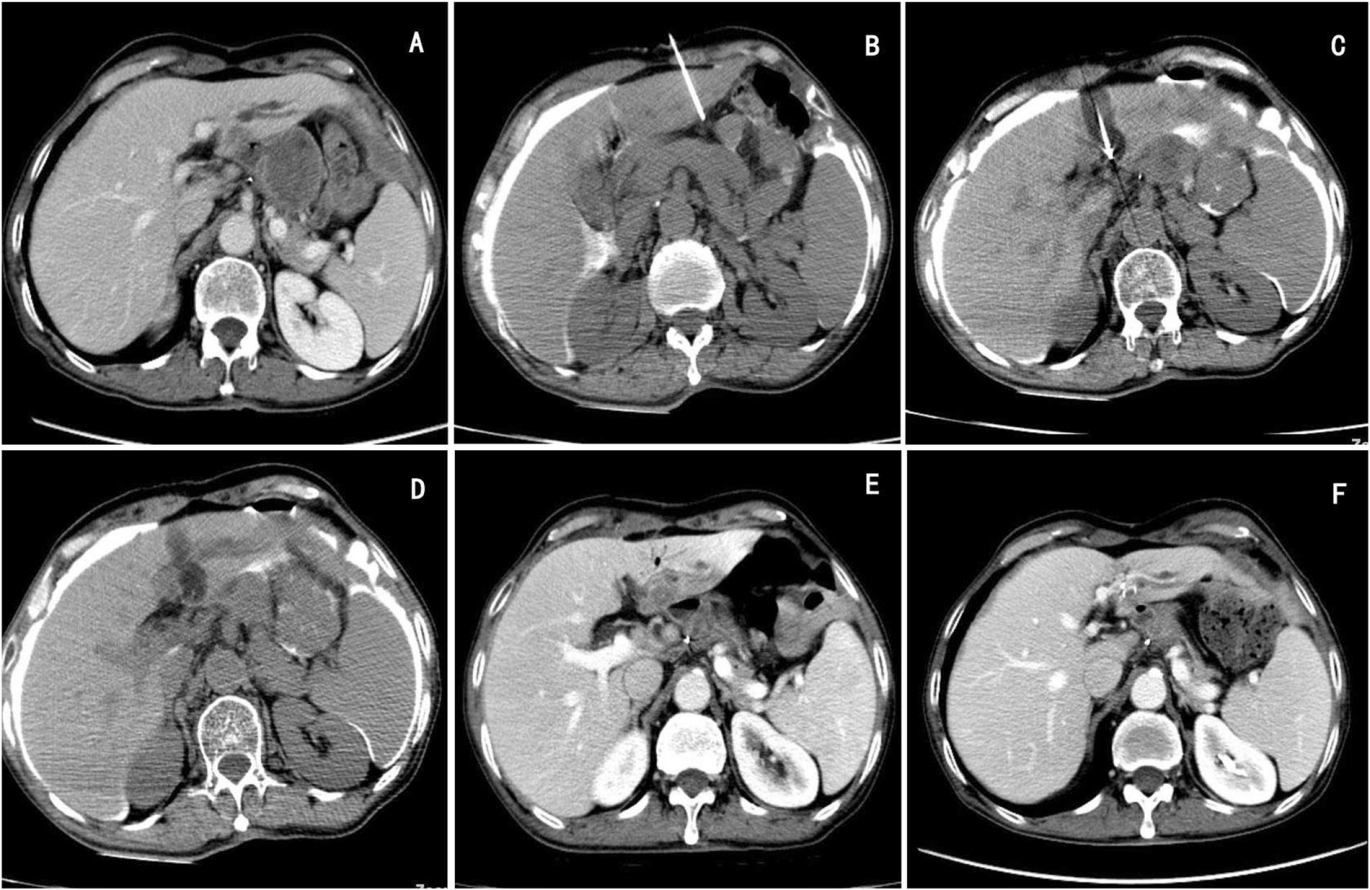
Esophageal carcinoma with liver metastases in a 59 year old man. (A) Contrast-enhanced CT demonstrated a low density nodule adjacent to portal vein in left lobe of liver, and there is not clear limit between the nodule and the posterior gastrointestinal tract. (B) A 20-gauge introducer needle was inserted into the hepatogastric space under CT guidance, and then a sufficient amount of artificial ascites was injected. (C) and (D) Cryoablation was applied under CT guidance. (E) Contrast-enhanced CT showed no injury in the adjacent gastrointestinal tract after three days follow-up. (F) Contrast-enhanced CT showed the focus was shrunk with no signs of recurrence after three months followed up.
